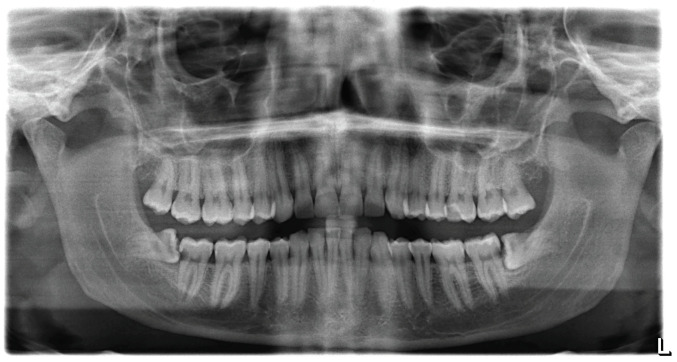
Initial panoramic radiograph of the patient with both lower wisdom teeth mesially inclined, partially impacted, and in strict contact with the distal wall of the adjacent tooth affected by dental caries.
